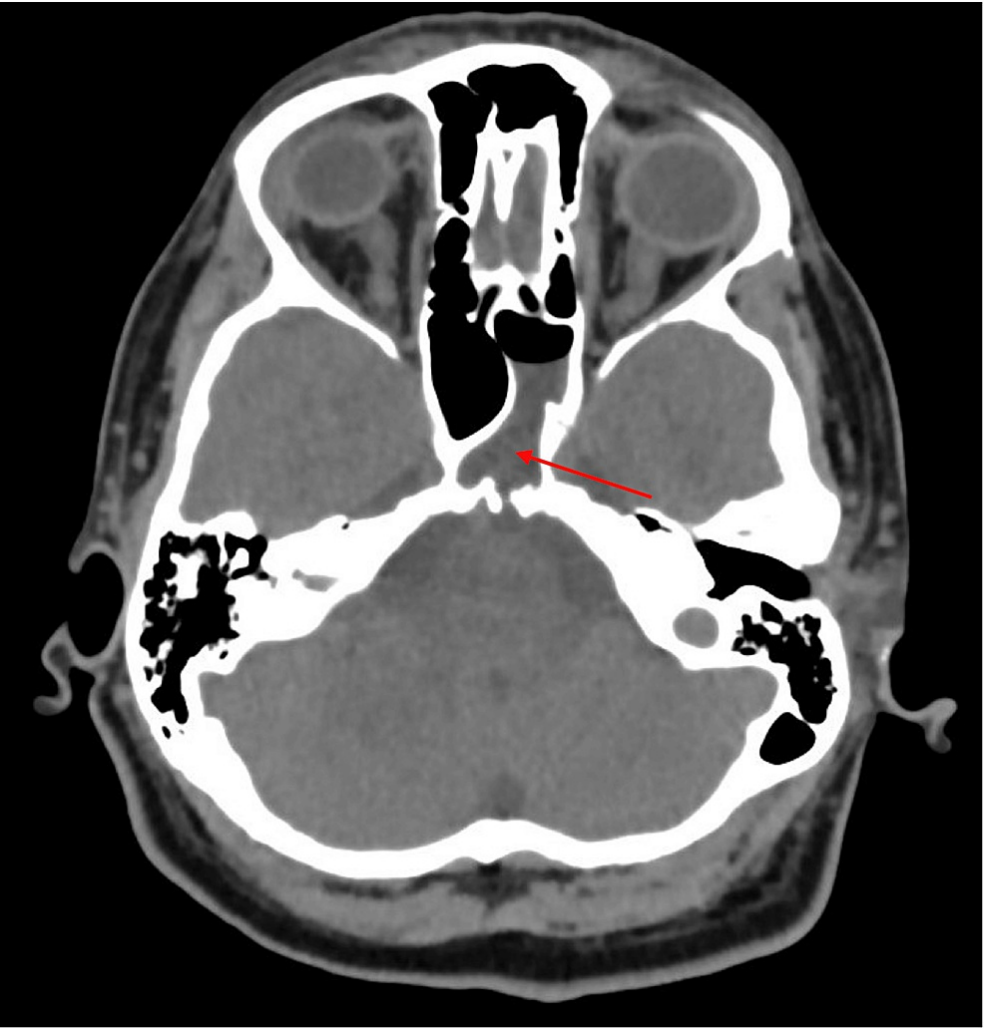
CT intracranial angiogram at hospital admission The red arrow highlights sphenoid sinus contrast extravasation concerning for a CSF leak.
